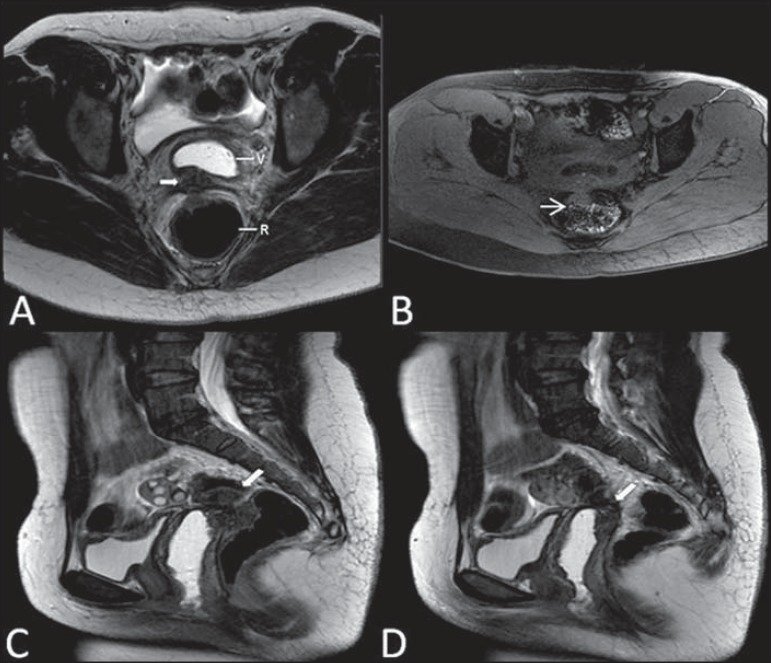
Multiplanar MRI T1-weighted (B) and T2- weighted
